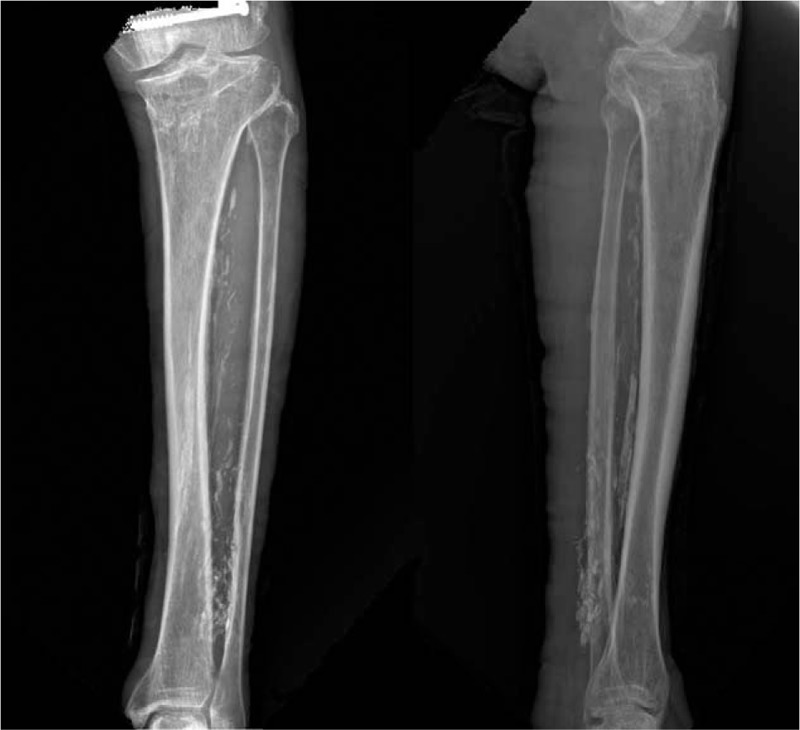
Postoperative radiographs of the left leg showing decreased radiopaque mass.
